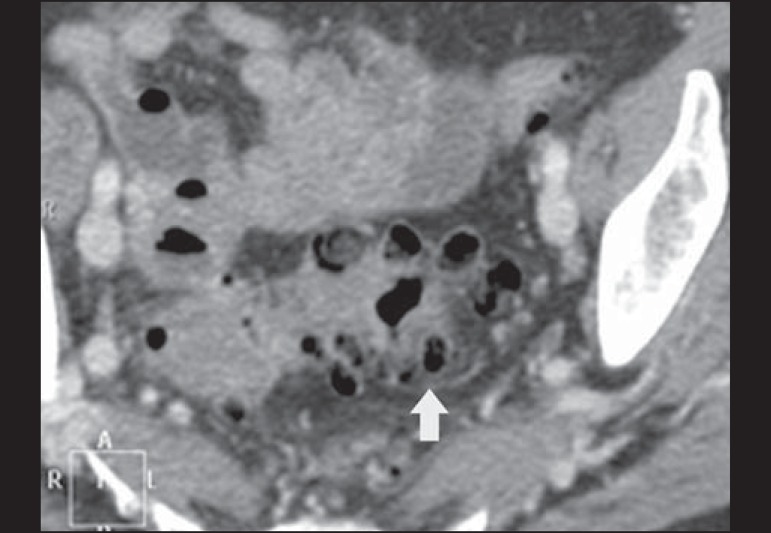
Hinchey stage 0. Intravenous contrast-enhanced abdominal CT, in the axial
